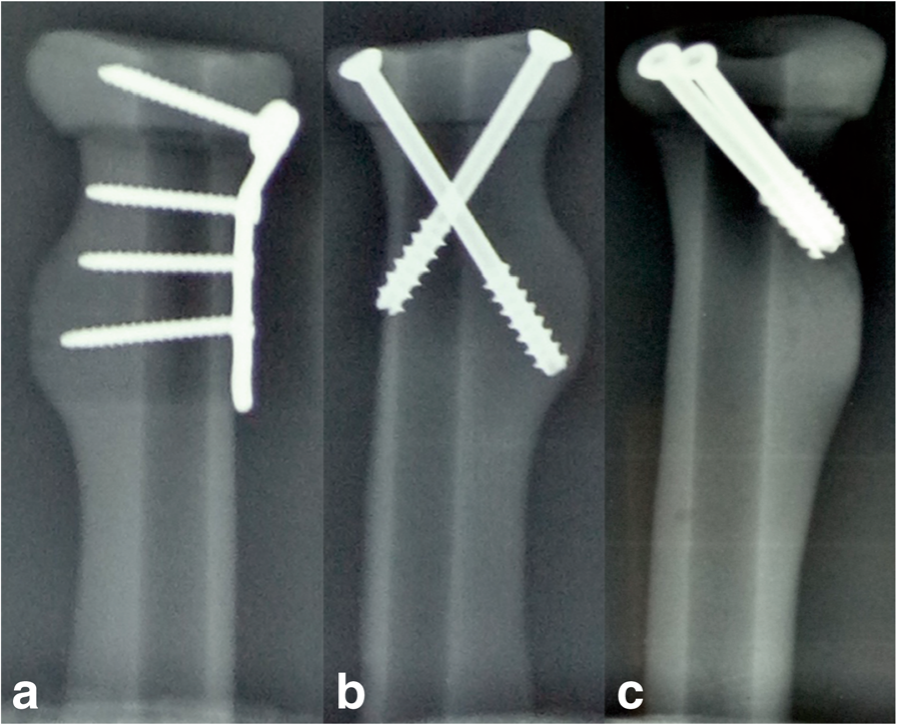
Radiographs of the plate (a), crossed screw (b), and parallel screw (c)
